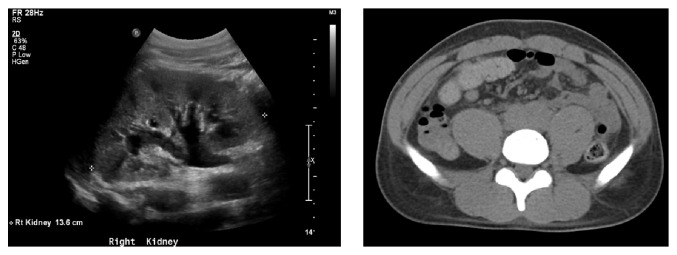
Initial imaging studies. Left: renal ultrasound at presentation revealed moderately enlarged kidneys with increased renal cortical echogenicity and mild bilateral hydronephrosis. Right: abdomen and pelvis computed tomogram without contrast revealed mild inflammatory changes without lymphadenopathy.
